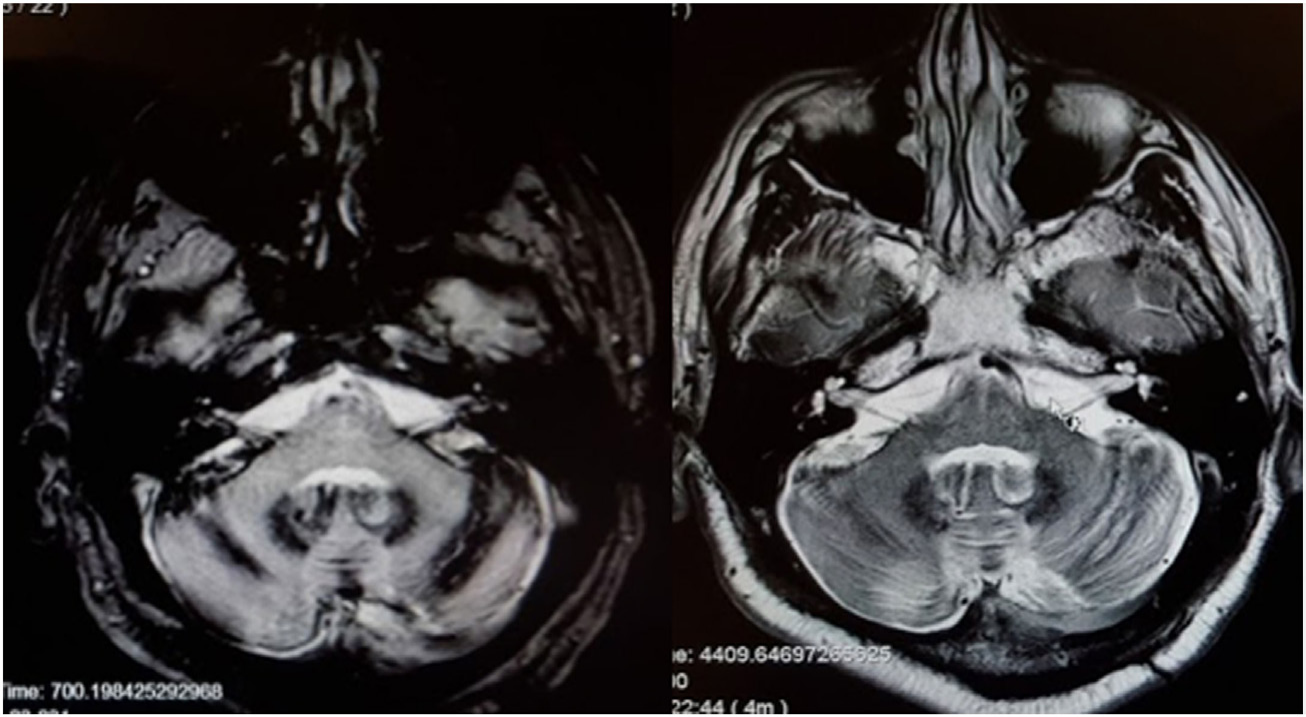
MRI of a patient with siderosis showing hemosiderin deposition along the vestibulocochlear nerves. The image on the left is an axial gradient-echo (GRE) T2*-weighted sequence. The image on the right is an axial T2-weighted image. Both figures are through the internal auditory canals. The study was performed on a 1.5-Tesla strength MR.
